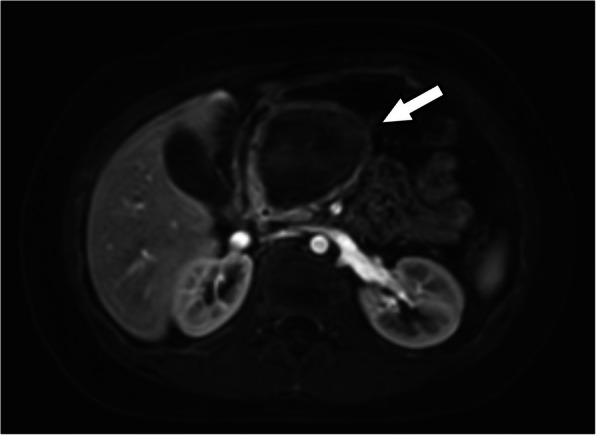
MRI with contrast. According to MRI data, there was visualized a heterogeneous cystic formation, 55 × 51 × 48 mm, at the level of the head and body of the pancreas
